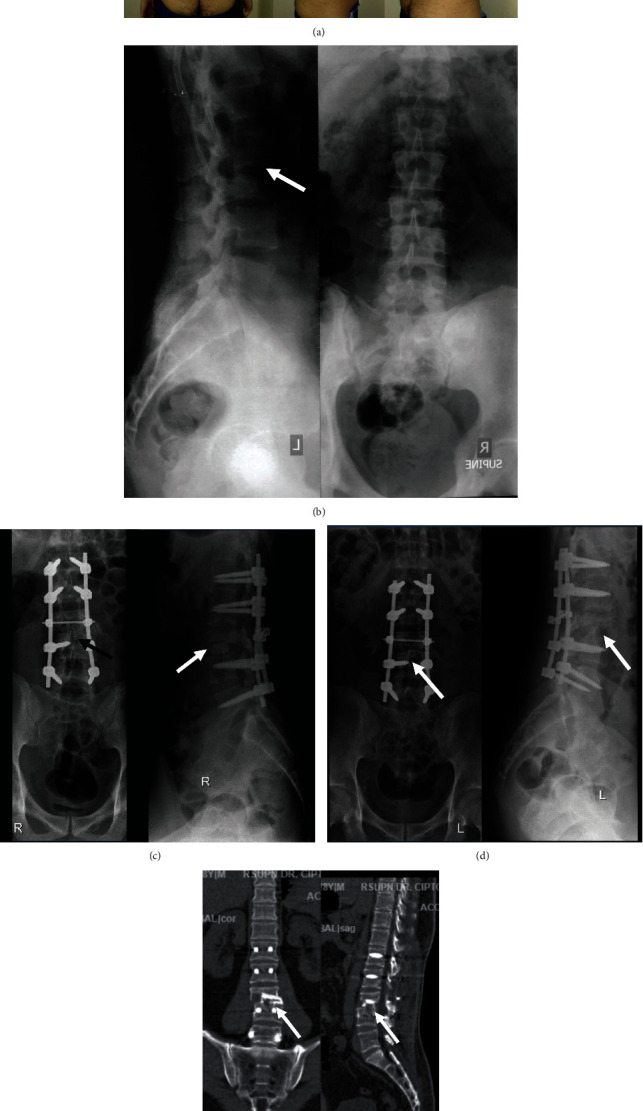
A 27-year-old male (case 2). (a) Preoperative clinical picture showed hyperlordosis. (b) Preoperative radiography showed defect on the anterior column around 55% of the corpus vertebrae. (c) One-month postoperative radiography showed new bone formation that filled around 35% of the corpus vertebrae. (d) Three-month postoperative radiography showed new bone formation that filled around 60% of the corpus vertebrae. (e) Six-month postoperative CT scan showed 60% of new bone formation on the left posterior lateral of the corpus vertebrae. Sites are shown using white and black arrows.
